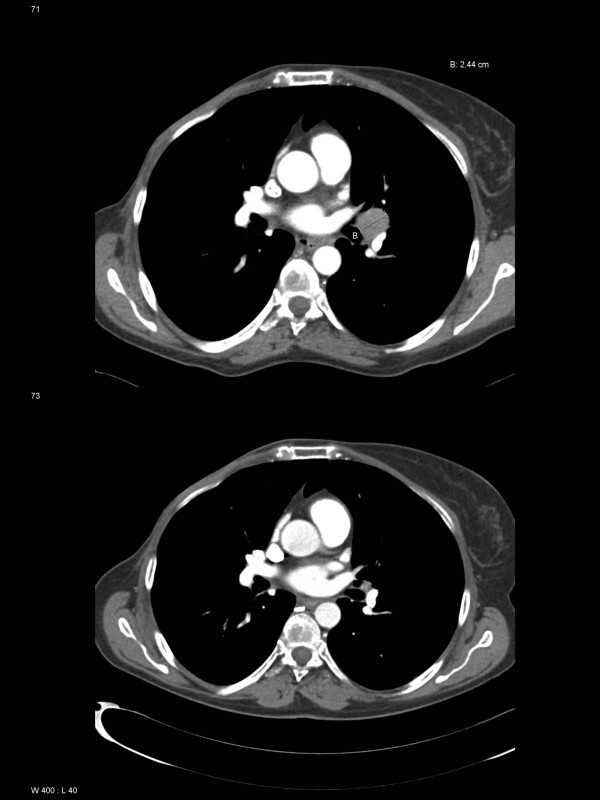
Baseline and follow-up lung imaging. Computed tomography scans of the chest at diagnosis of metastatic breast cancer demonstrate a left hilar mass of 2.44 cm diameter (upper image, August 2006). In November 2007, a continued partial remission was found (lower image).
